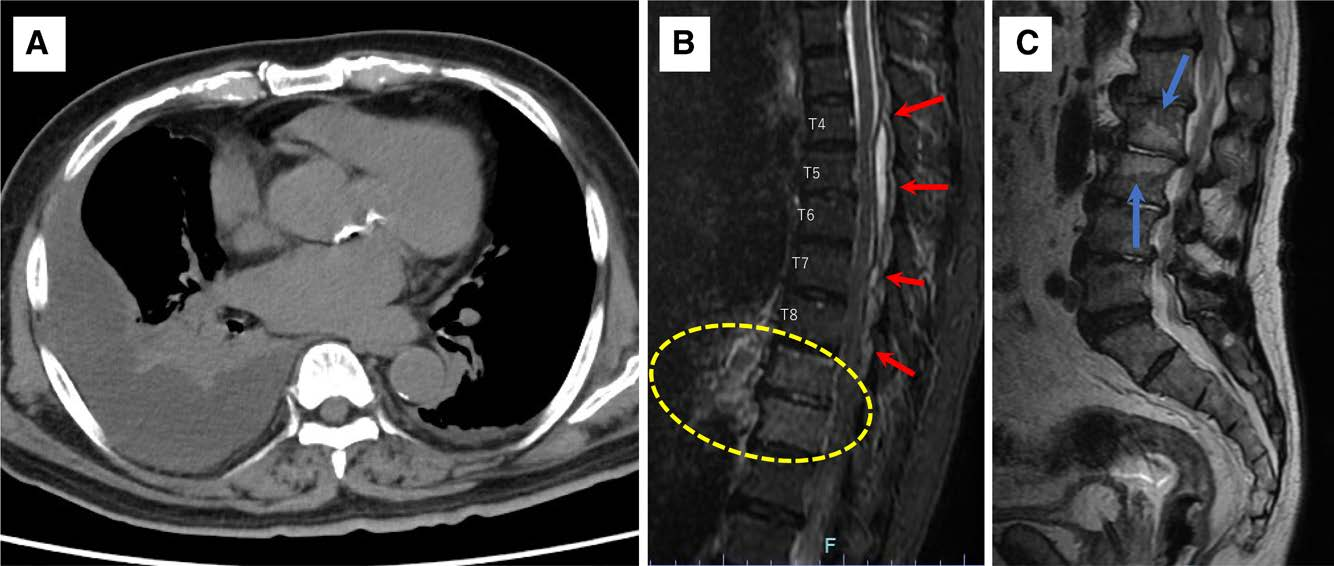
Image of the abscess and spondylodiscitis at intensive care unit transfer. (A) Chest computed tomography image showing dense right pleural effusion. (B) MRI (STIR sequence) showing abscess formation in the T4–8 regions (red arrowhead); yellow dotted circles denote spondylodiscitis and edematous changes in the surrounding soft tissues at the T9–10 regions. (C) MRI (T2-weighted sequence) showing spondylodiscitis between the L2–3 regions (blue arrowheads). MRI = magnetic resonance imaging, STIR = short tau inversion recovery.
